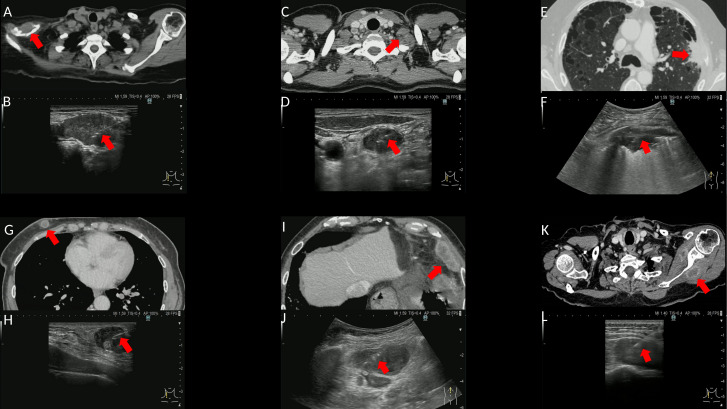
Axial CT images and corresponding US-NAB images of various metastatic and primary lung lesions. Panels (A,B): bone metastasis (arrows); Panels (C,D): supraclavicular lymph node metastasis (arrows); Panels (E,F): pulmonary primary tumor (arrows); Panels (G,H): subcutaneous nodular metastasis (arrows); Panels (I,J): pleural metastasis (arrows); Panels (K,L): periscapular muscular metastasis (arrows).
